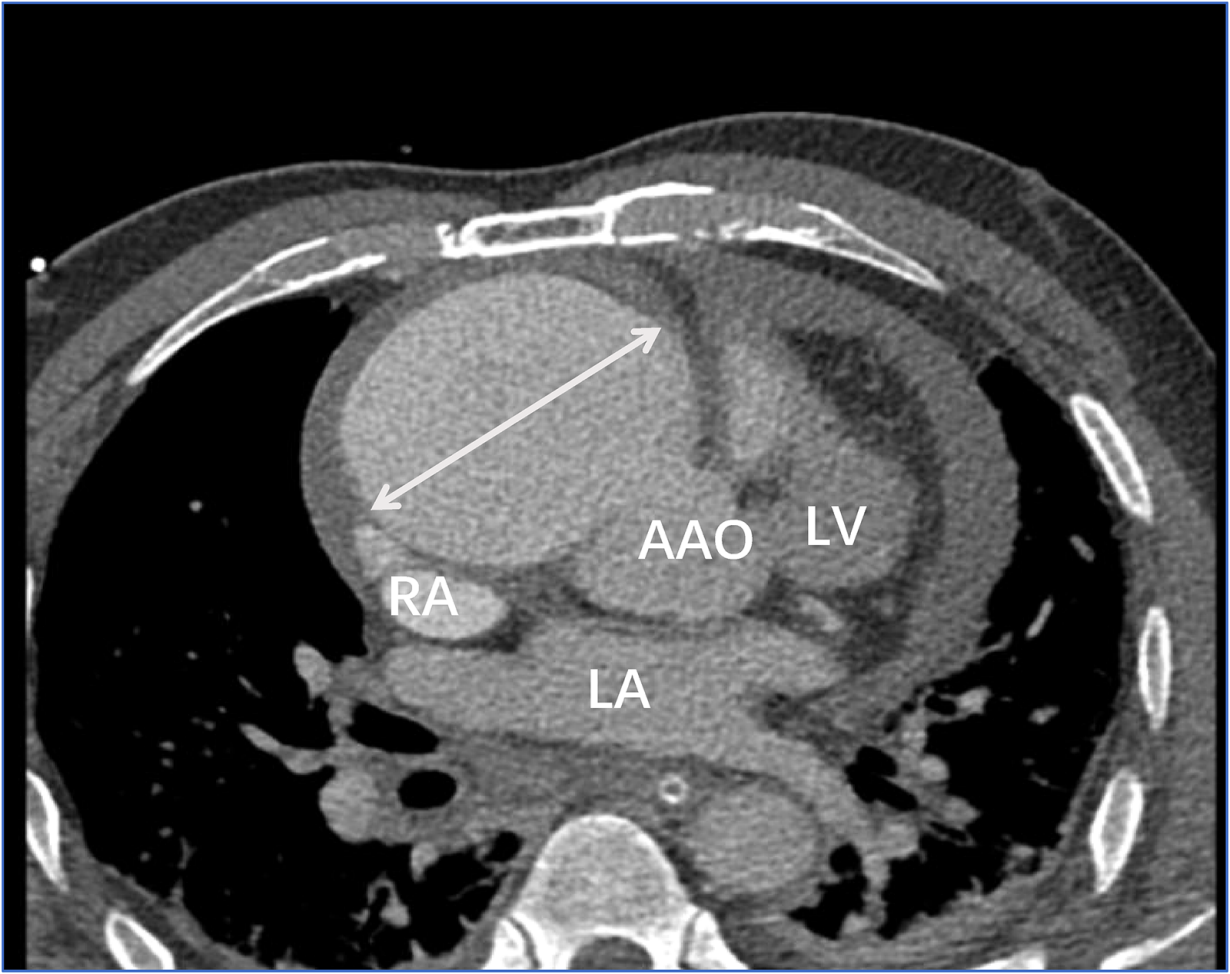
Contrast-enhanced computed tomography angiography confirmed a spherical dilatation (6.0 cm × 7.5 cm) in the right anterior aspect of the ascending aorta (white arrow). LA, left atrium; LV, left ventricle; RA, right atrium; AAO, ascending aorta.
